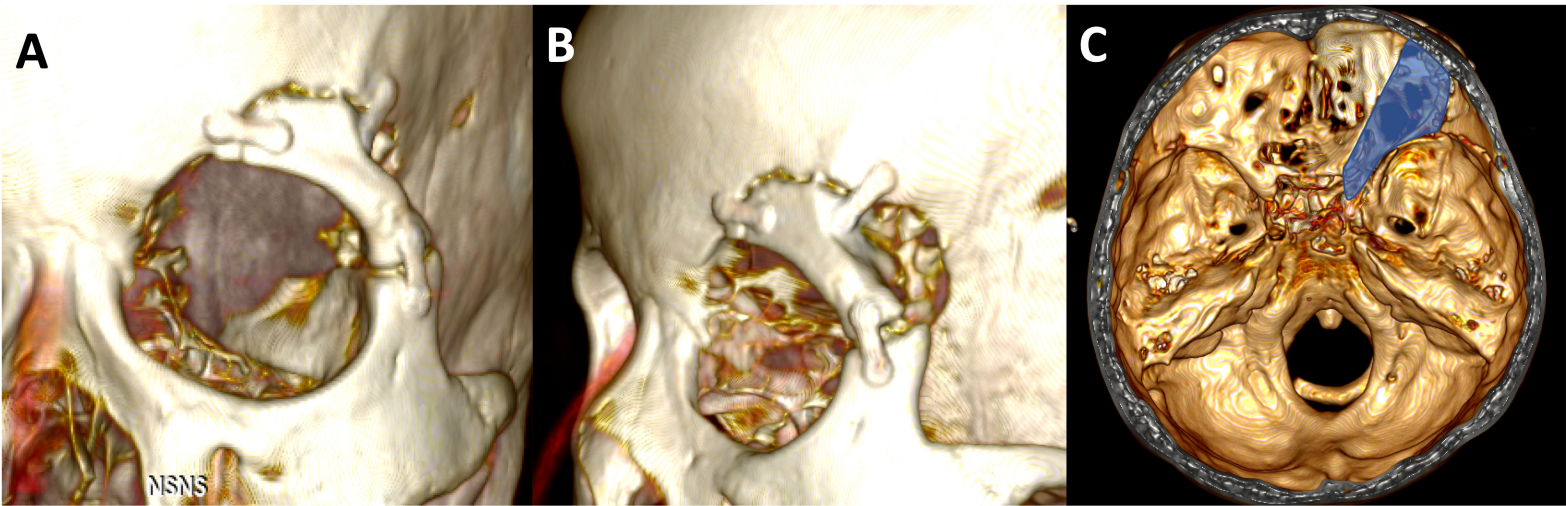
(A, B) 3D reconstruction images from postoperative CT scan of the patients underwent extradural anterior clinoidectomy using ETOA with SLOR osteotomy. Superior-lateral orbital rim was resected in one-piece and fixed firmly with miniplates. (C) The skull defect including ACP is showed as the blue highlighted area. ACP, anterior clinoidal process; ETOA, endoscopic transorbital approach; SLOR, superior-lateral orbital rim.
